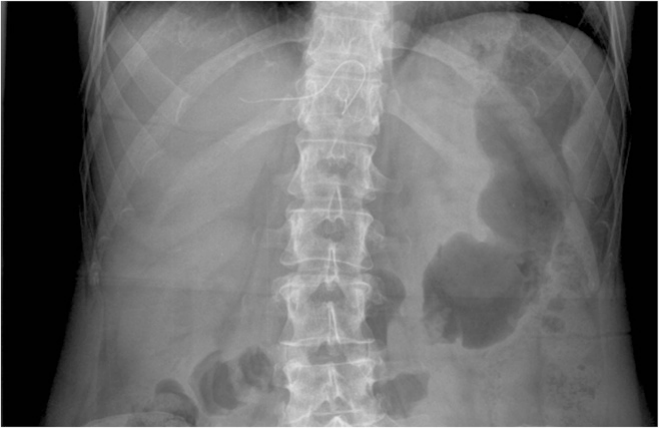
Retained epidural fragment at abdominal X-ray. A pre-operative abdominal plain X-ray showing the retained epidural fragment at T11-12 level. The identification of the catheter was repeatedly missed over the previous 12 years. Note the significant bowel distension related to the patient’s underlying disease for which the x-ray was being taken
